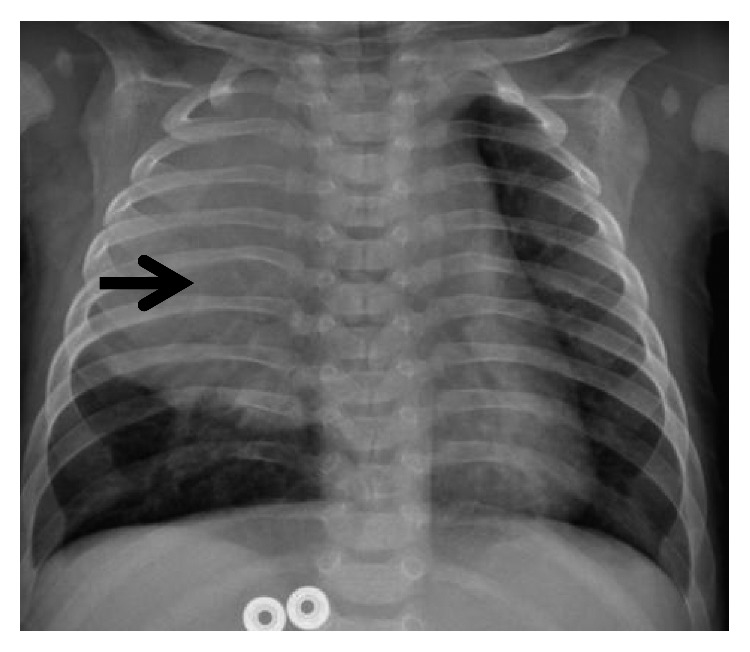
Chest X-ray: RUL infiltration and enlarged intercostal space in level T6-T7 on the same side.
